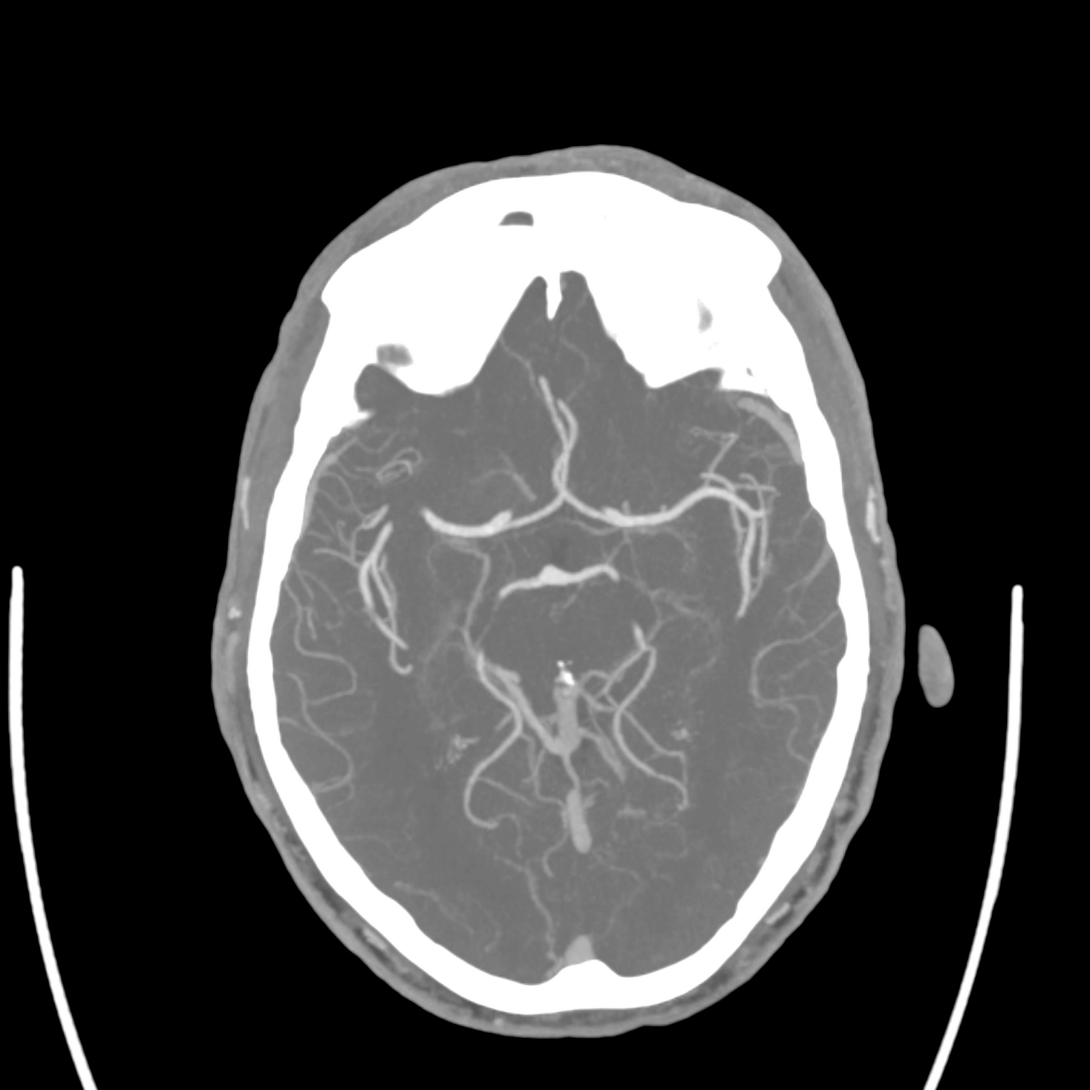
Normal computed tomography angiography without evidence of vessel occlusion or stenosis.
